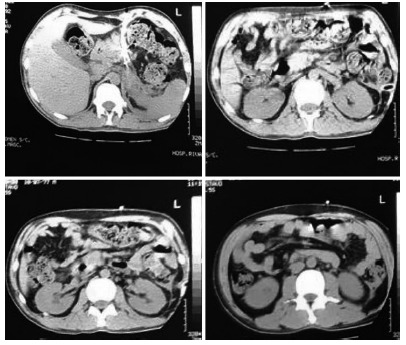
Reduction of peri-pancreatic collection at follow-up CT scan
